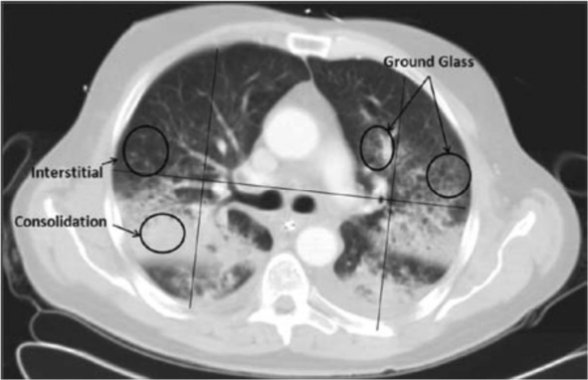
Example of radiologist’s score findings in chest computed tomography scan slice [34]
